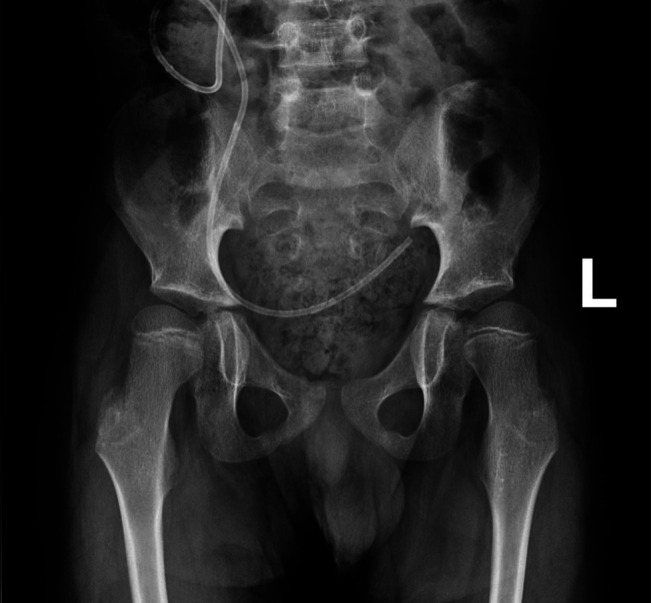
Prehospital admission anterior-posterior radiograph of pelvis indicating preexisting hip subluxation with a Reimers migration index of 40%.
